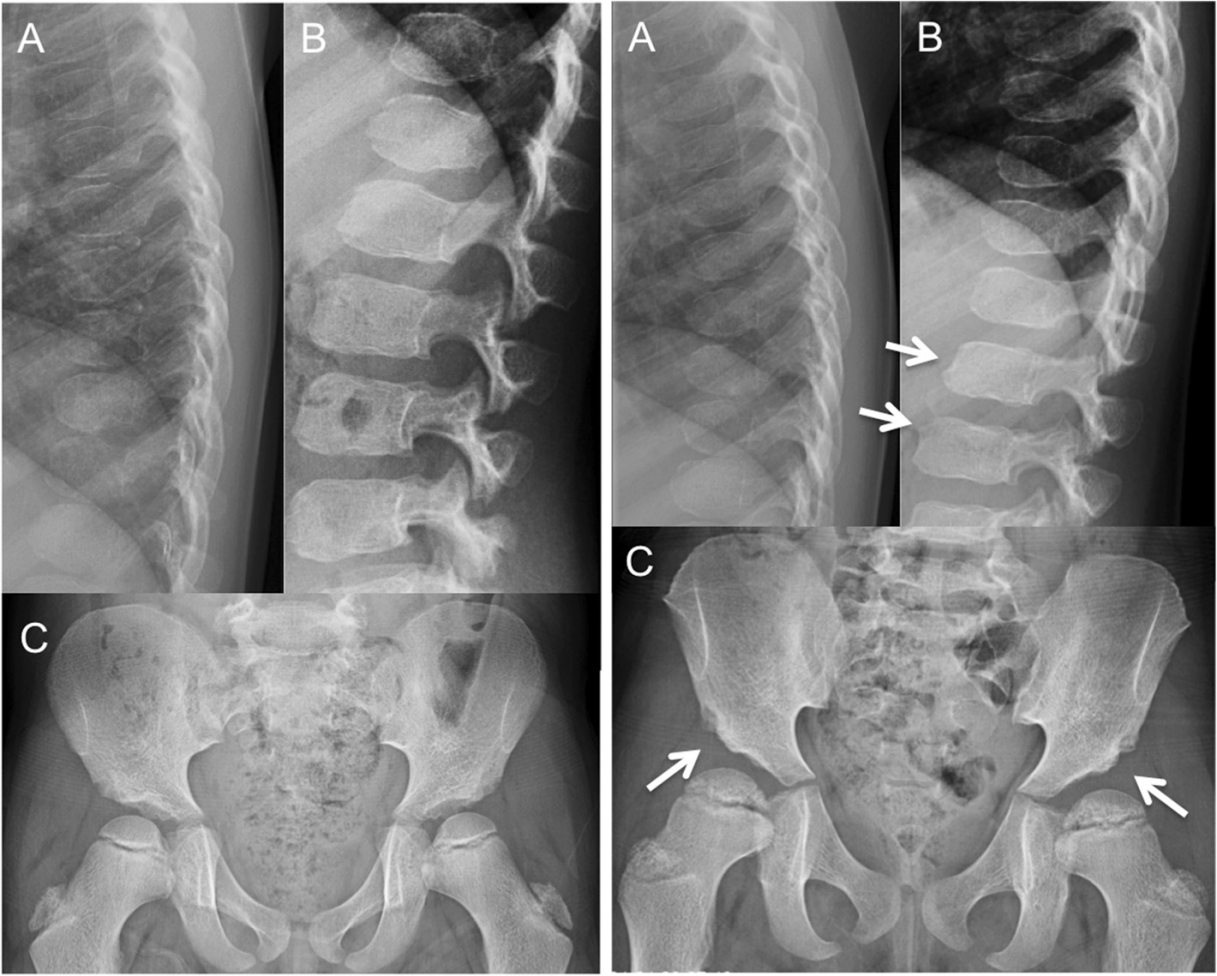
X-ray of pelvis, thoracic and lumbar spine. Left panel: Patient 1 (male, age 6.5 years) had a possible biconvex L1-vertebrae, although no obvious spinal pathology (A, B). The hips are normal for age (C). Right panel: Patient 2 (female, age 5.2 years) shows slightly flattened lumbar vertebrae, a possible pre-stage of anterior “beaking” typical for MPS VI (B, arrows), and bilateral steep acetabular angles (C, arrows).
